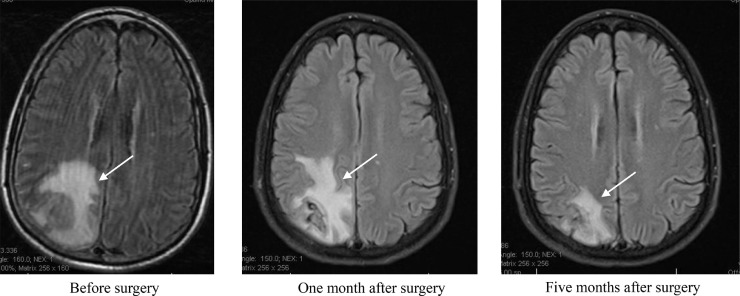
The MRI of the brain. The first MRI (before surgery) with noncontrast confirmed the right parietal rim-enhancing lesion. The second and third MRI follow-up (with and without contrast) showed significant improvement in size and surrounding vasogenic edema. All the provided images are axial T2 Flair.
